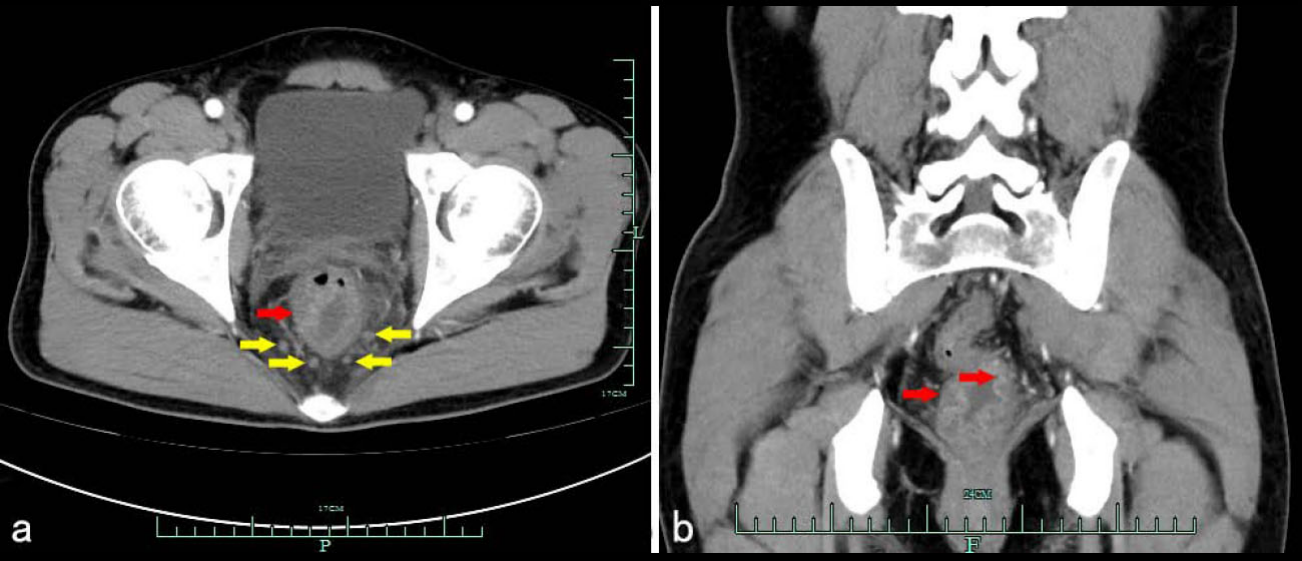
Pelvic Enhance CT in patients with CMV colitis: (a) axial scan; (b) coronal scan. CT scan showed Edema and irregular thickening of the rectal wall (red arrow), obvious enhancement of the mucosa (red arrow), and multiple enlarged lymph nodes around (yellow arrow).
